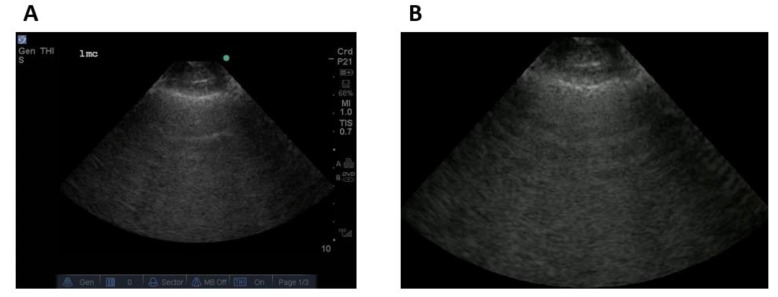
Masking of native ultrasound image (A) resulting in a frame consisting of only the ultrasound image without extraneous screen markings (B).
